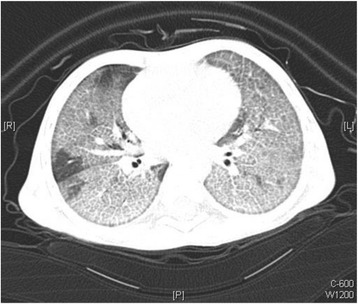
Computed tomographic scan of the chest showed ground-glass opacification and interlobular septal thickening (crazy paving pattern)
